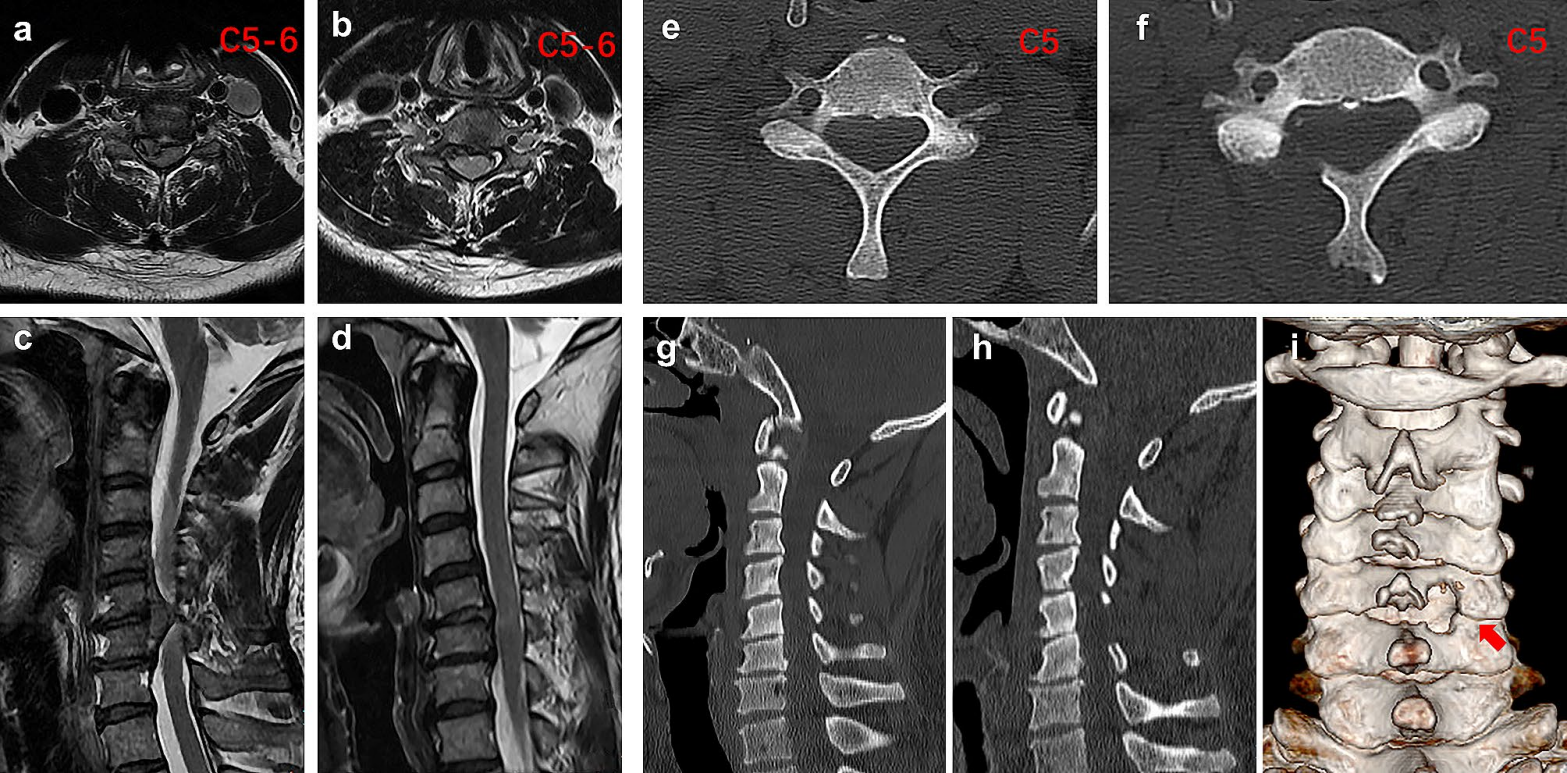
Pre- and postoperative magnetic resonance imaging (MRI) and computed tomography (CT) scans. (a and c) Preoperative axial and sagittal MRI scans. At the left C5-C6 level, the soft disk protrudes laterally. (b and d) Postoperative axial and sagittal MRI scans showing that the protruded disk was removed. (E and F) Preoperative and postoperative axial CT images of the cervical spine. (G and H) Preoperative and postoperative sagittal CT images of the cervical spine. (I) Postoperative 3-dimensional CT image
